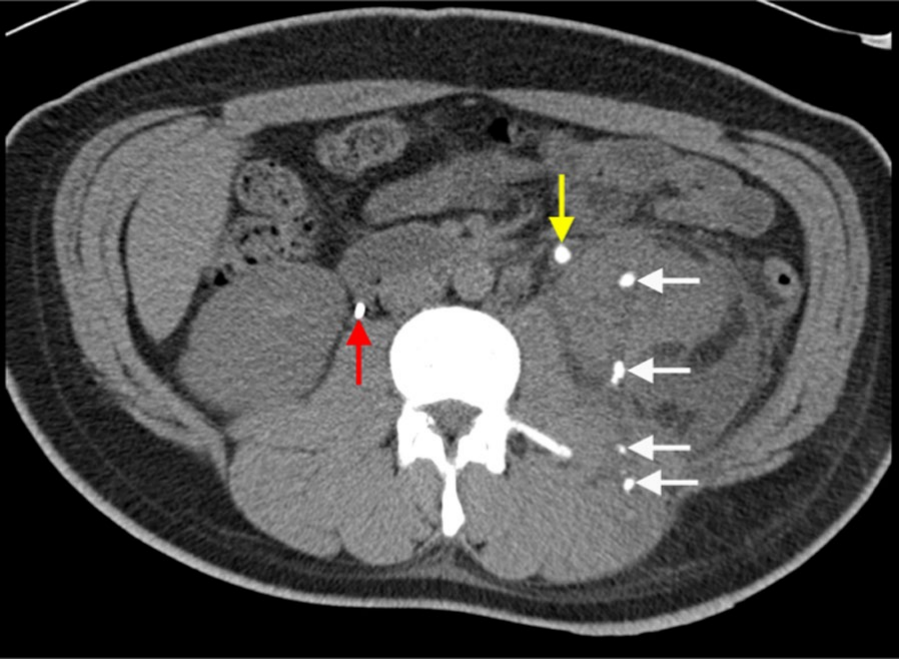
Unenhanced CT image of migration of renal calculi (white arrows) into the left percutaneous nephrolithotomy tract and a small left perinephric urinoma post-PCNL of a left staghorn calculus. There is a inferior migration of the ureteric calculus within the left proximal ureter (yellow arrow). A right-sided ureteric stent is in situ (red arrow)
